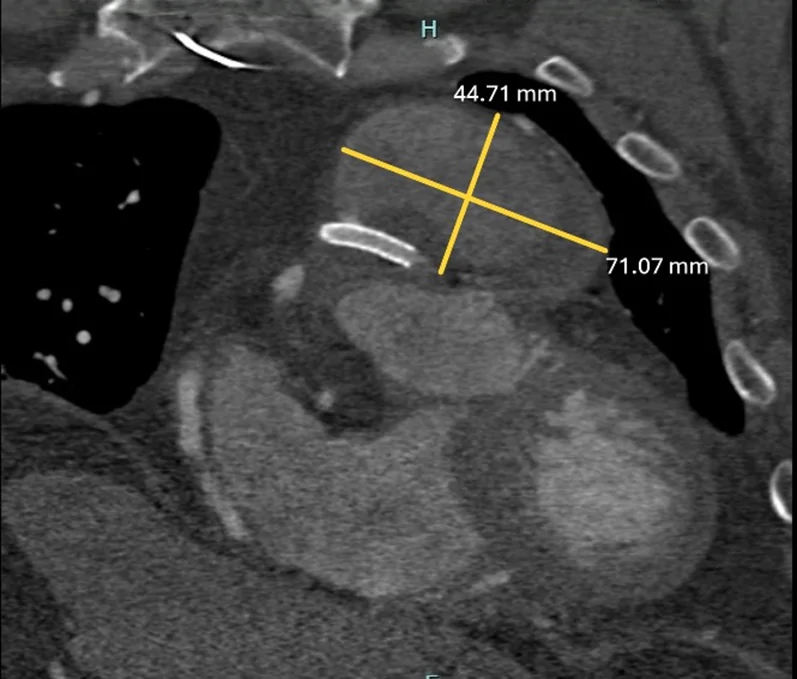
Coronary Computed Tomography Angiogram Shows A Large Pseudoaneurysm of the Saphenous Vein Graft
